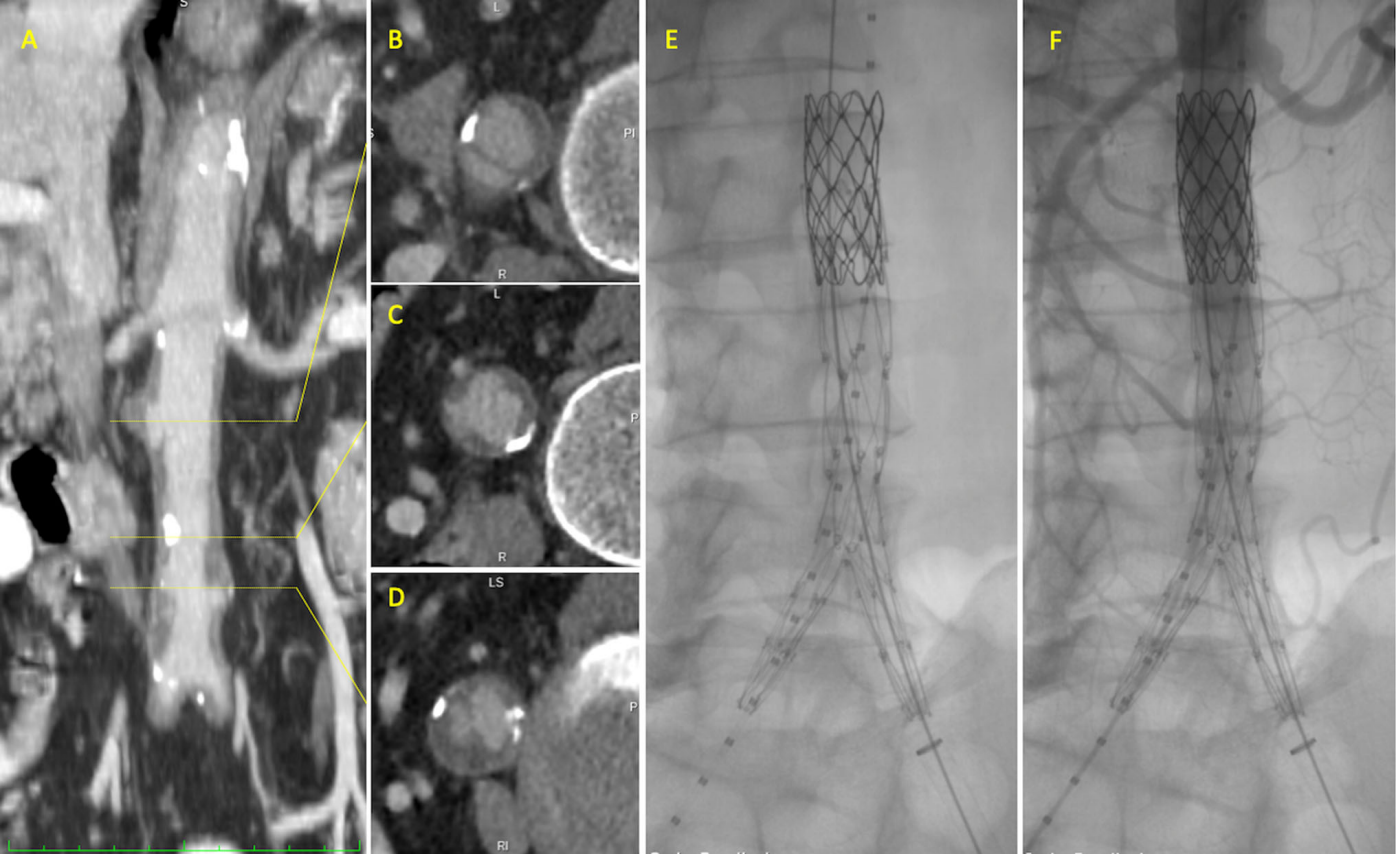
Preoperative CTA MIP showing overall infrarenal acute aortic syndrome (a). Infrarenal aortic dissection (b), intramural hematoma (c) and penetrating aortic ulcer (d), intraoperative arteriogram after unibody stent-graft deployment and proximal extension (e), and after contrast media injection (f)
